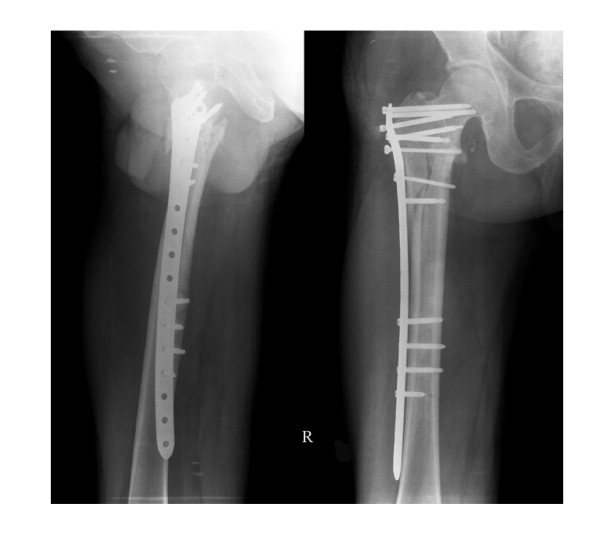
Lateral radiograph and AP radiograph at 4 weeks post fixation showing callus formation.
